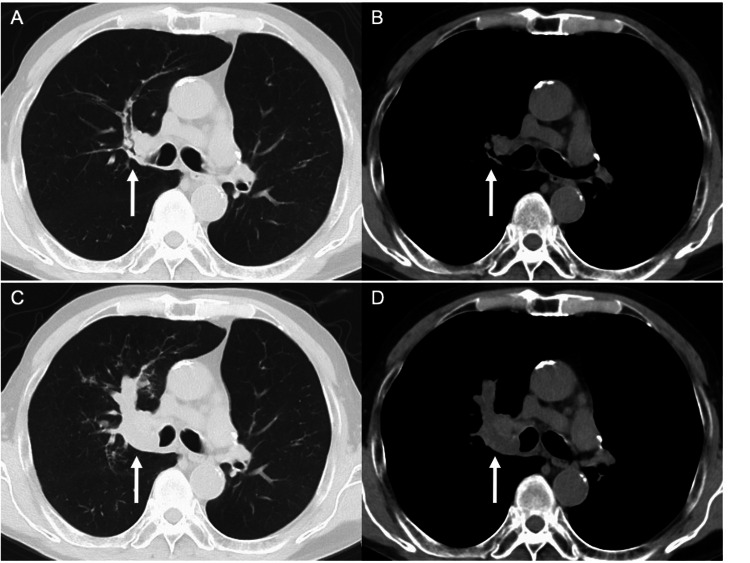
Chest computed tomography performed 14 months before admission shows thickening of the bronchial wall in the hilar region of the right upper lobe of the lung (white arrow) (A, B), which evolved into an irregular mass extending into the bronchial lumen of the right upper lobe (white arrow) two months before admission (C, D).
